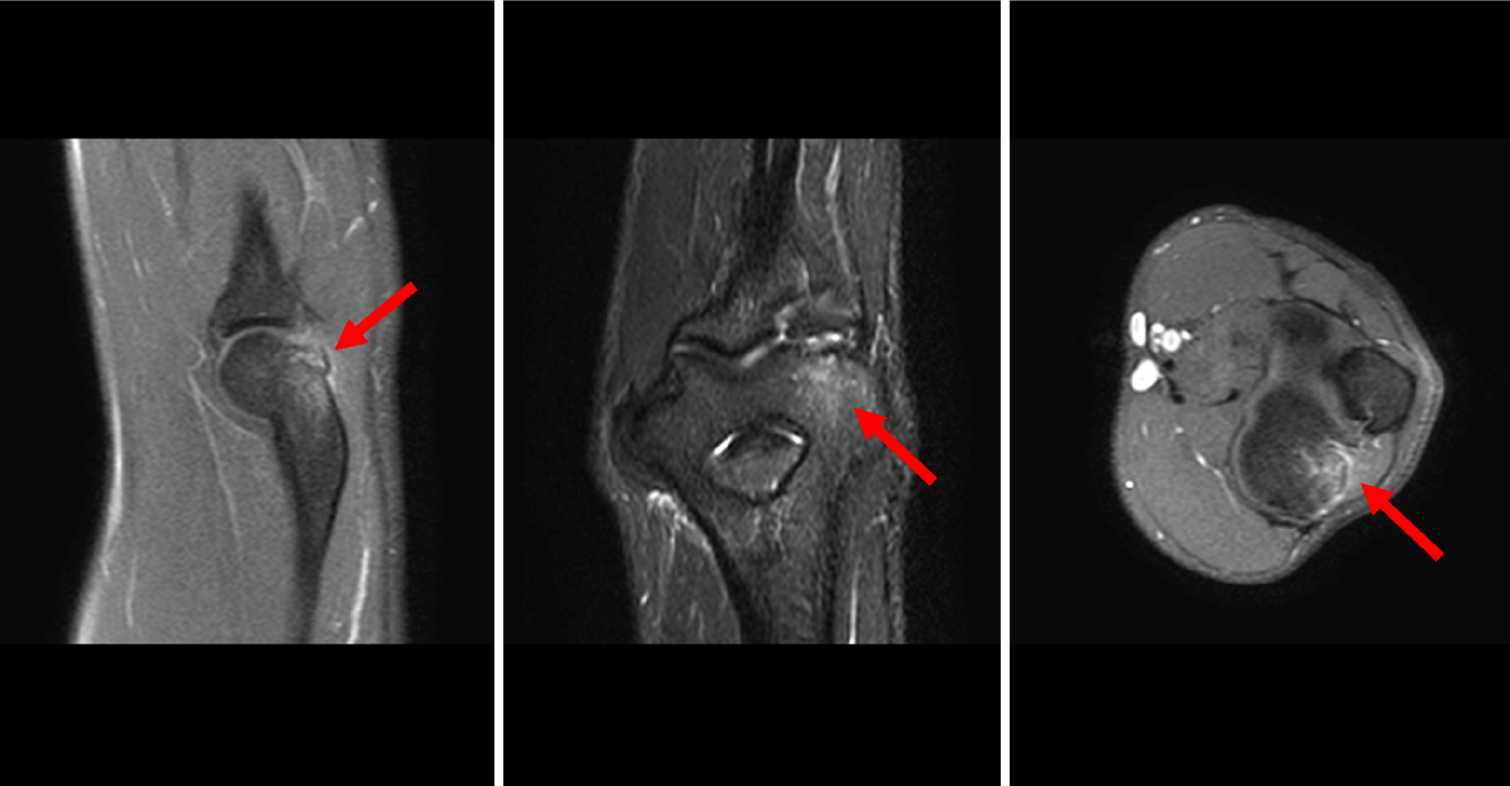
MR images of the right elbow of patient no. 4 at first presentation (the arrow marks the ossification)
